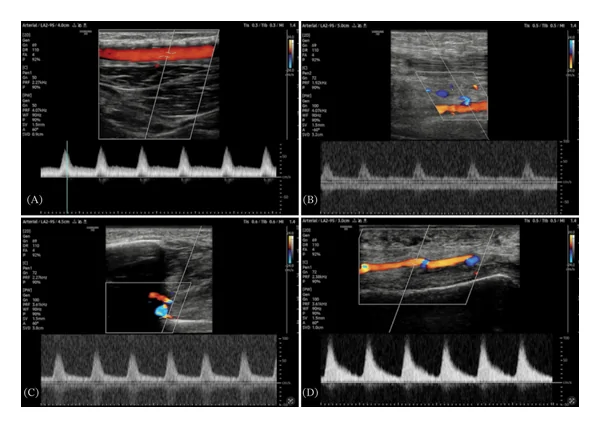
Representative duplex ultrasound surveillance showing sustained patency of the PTFE–ATA bypass and the controlled heel‐site bypass‐to‐vein vent without hemodynamically significant stenosis or excessive shunting. Labels: (A) PTFE graft; (B) anterior tibial vein takeoff/controlled heel‐site bypass‐to‐vein vent; (C) proximal ATA; and (D) dorsalis pedis artery.
